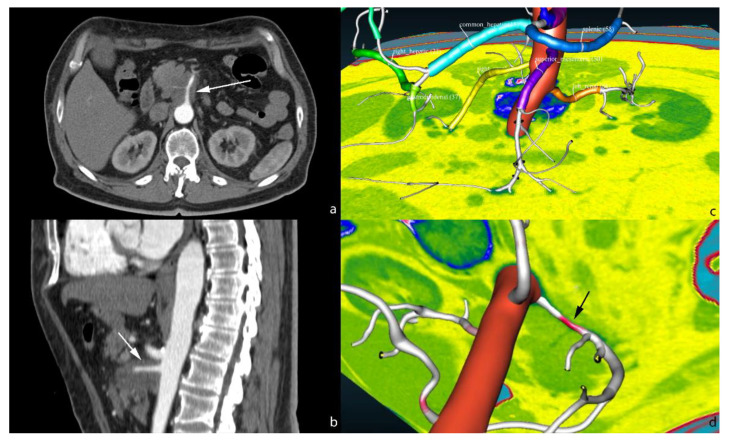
Axial (a) and sagittal (b) CT scan showing the infiltration of the superior mesenteric artery by pancreatic adenocarcinoma (white arrow). 3D view of automatic vessel labelling (c). Highlighting of the stenosis on the superior mesenteric artery in red (d, black arrow). The software may be configured to automatically output the length and entity of stenosis due to the tumour infiltration.
